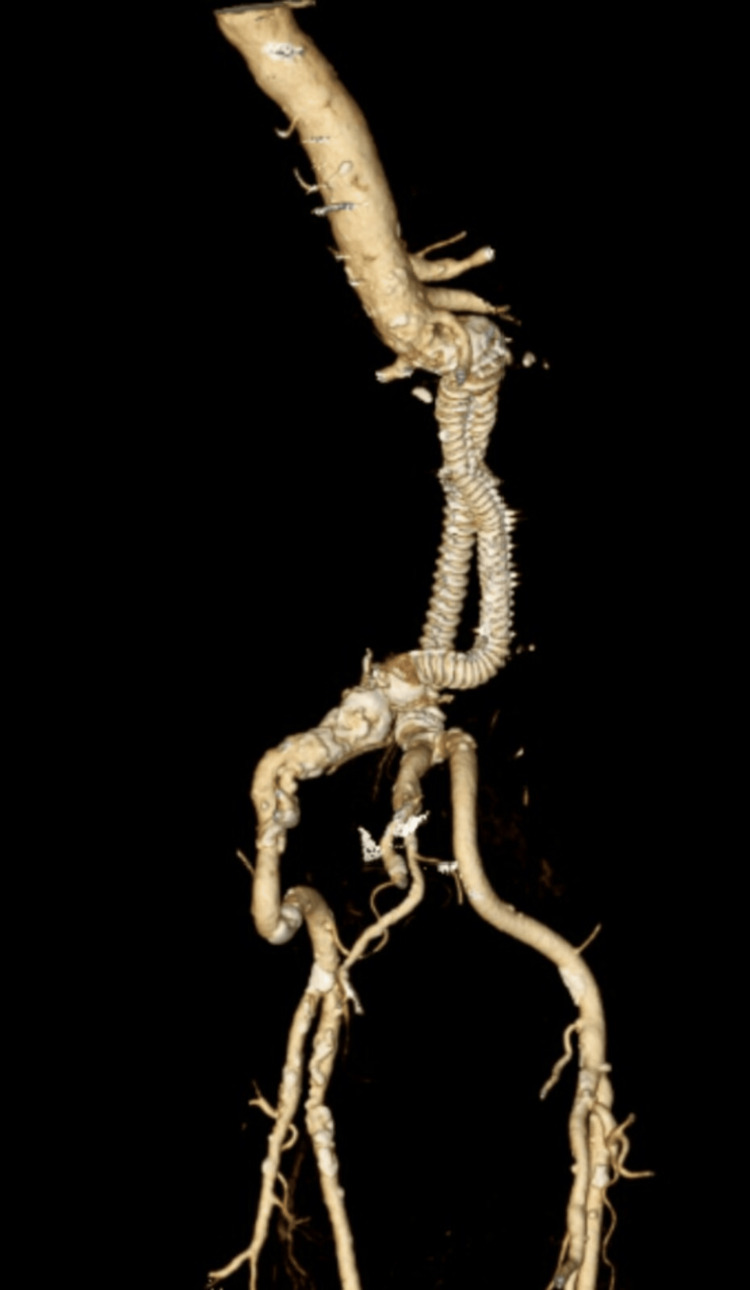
Follow-up CTA at 10 months post-EVAR Contrast-enhanced CTA demonstrating successful endovascular aneurysm exclusion. The Anaconda™ bifurcated endograft is well-positioned and fully patent, extending from the infrarenal aorta to the bilateral common iliac arteries. No endoleak is identified. Aneurysm sac measurements (139 × 124 × 116 mm) remain stable without significant interval change. No evidence of endograft migration, kinking, or limb compromise. CTA, CT angiography; EVAR, endovascular aneurysm repair
